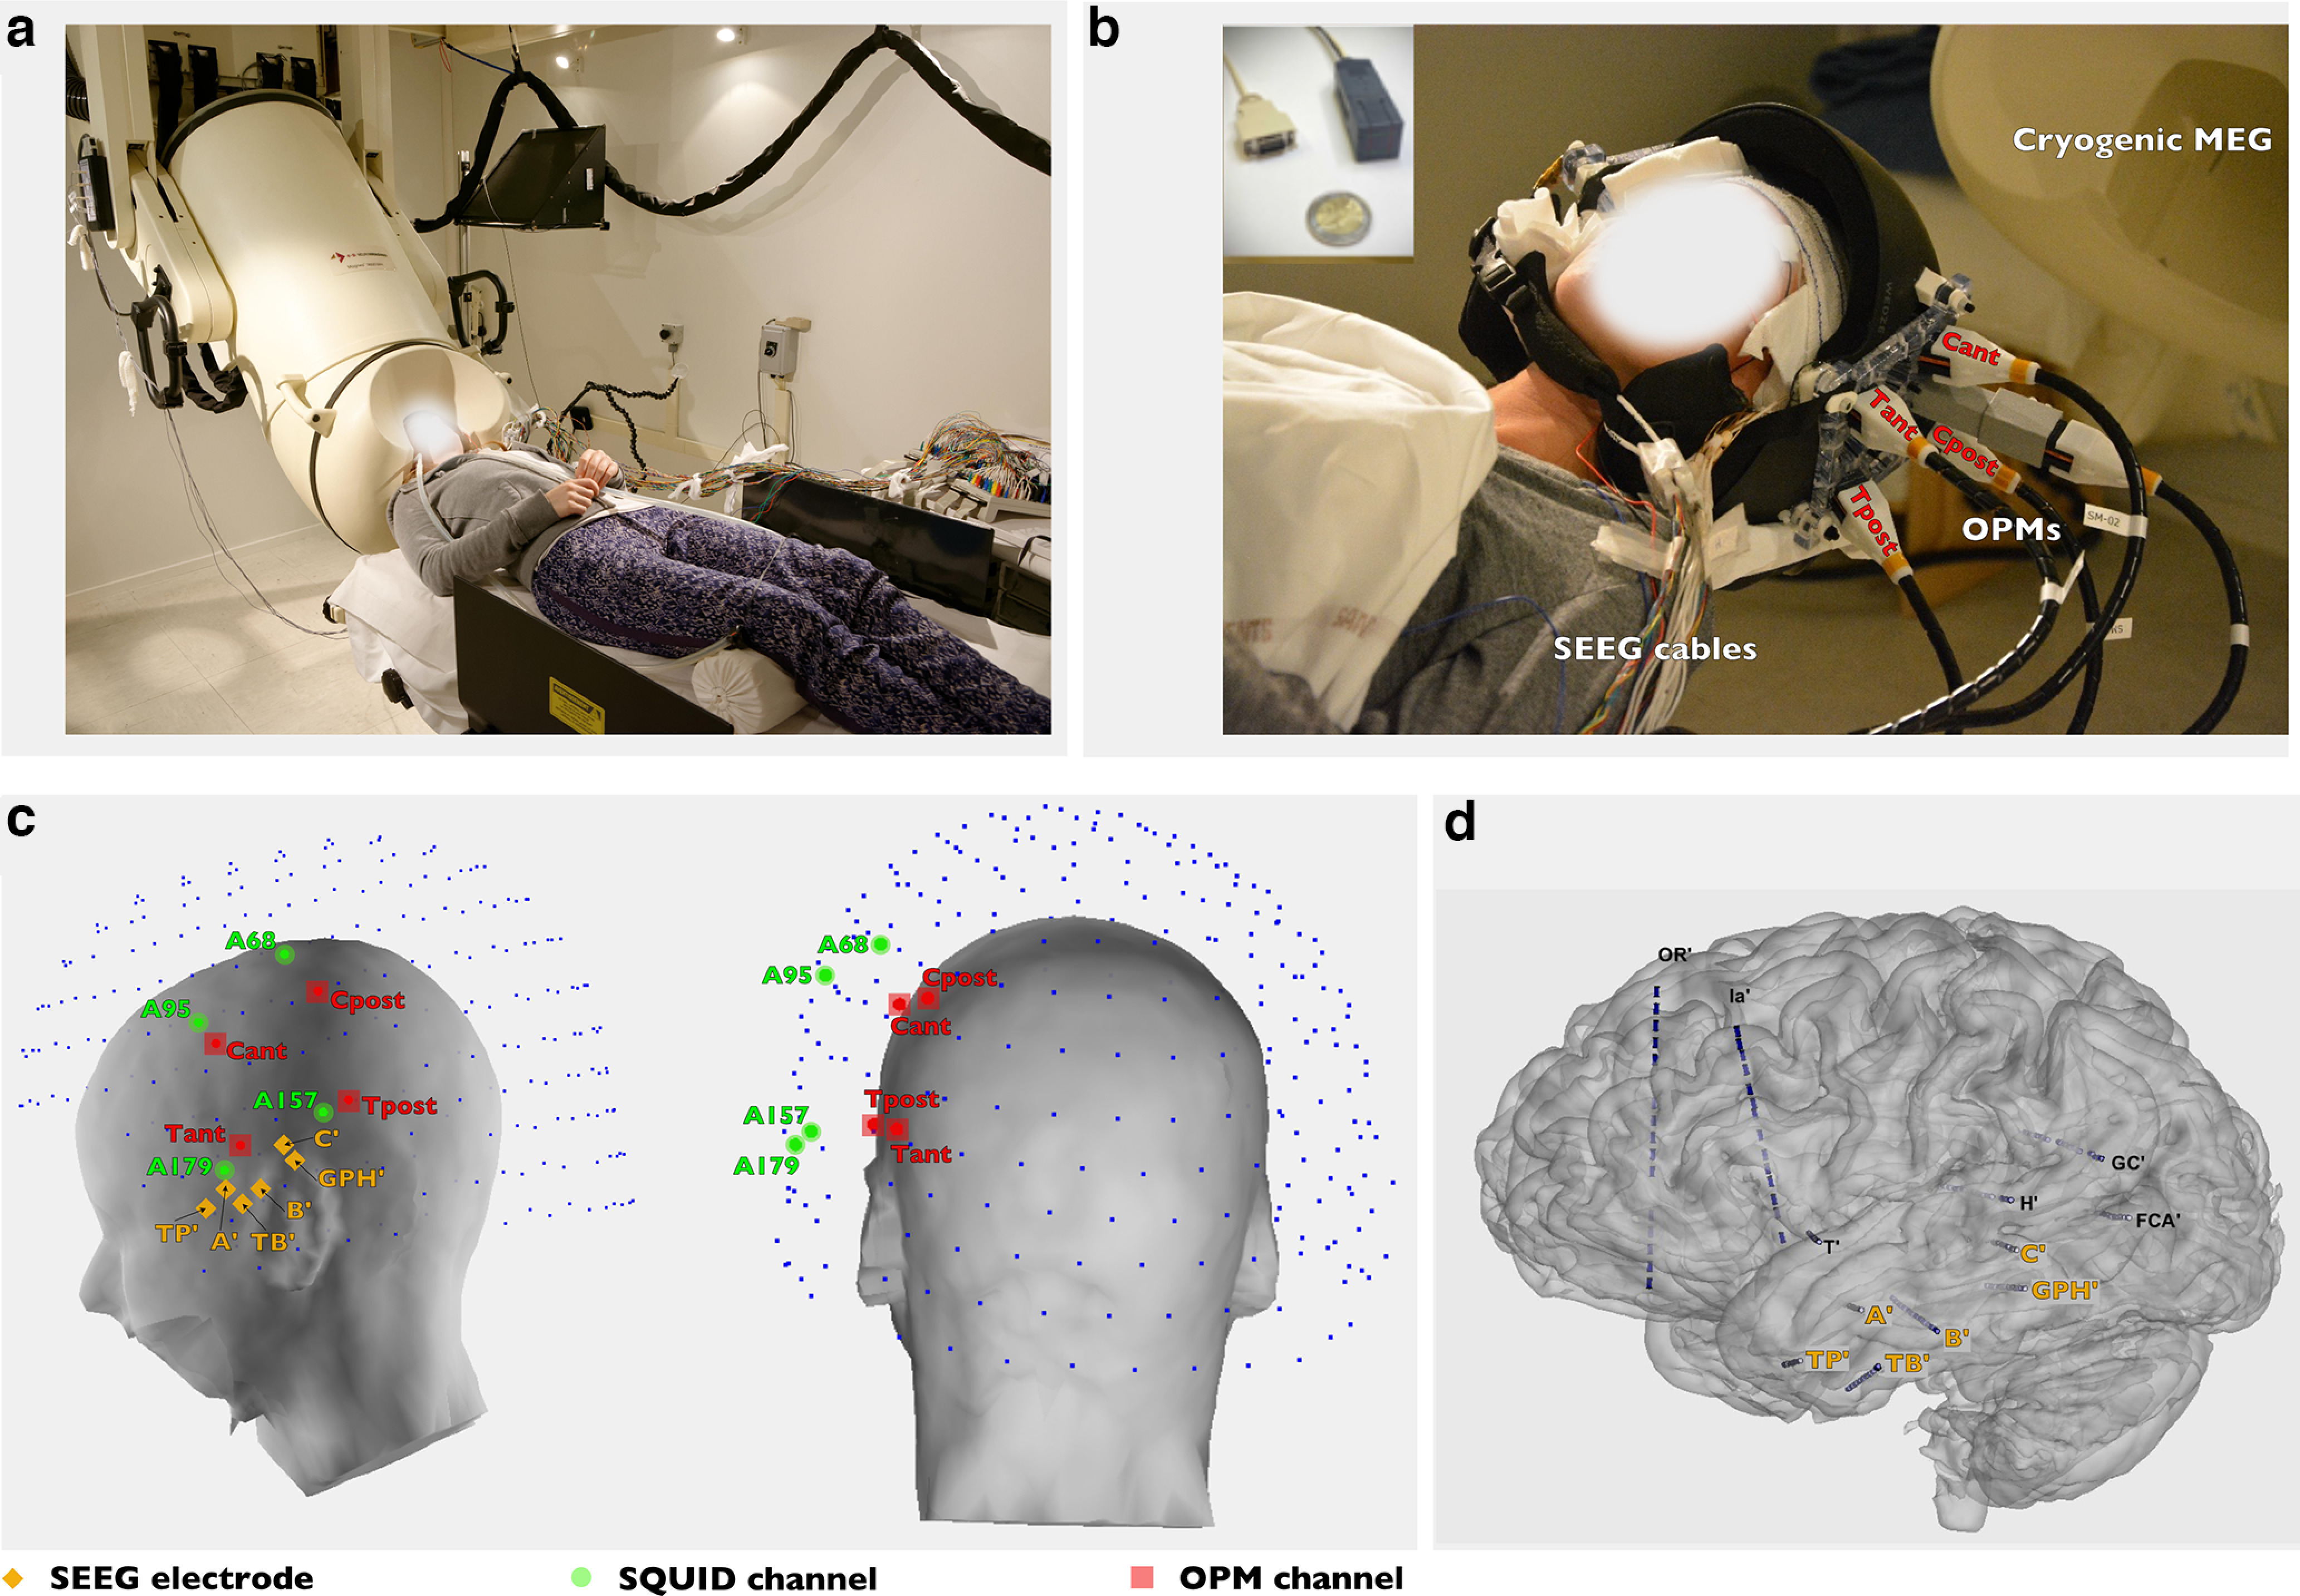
SQUID-MEG, ^4^He-OPM-MEG, and SEEG recording setup. *a*, Simultaneous SQUID-MEG/SEEG. The classic cryogenic MEG system, measuring 120 × 100 cm and weighing ∼300 kg with 265 SQUID sensors in a fixed array requiring subject immobility during data acquisition. *b*, Simultaneous ^4^He-OPM-MEG/SEEG: recording configuration composed of four sensors integrated into a wearable helmet placed on the scalp and in contact with the bandage covering the SEEG electrode inserts (the cables and connectors outside the helmet are visible). Insert, A photo of the ^4^He-OPM sensor. *c*, Three-dimensional reconstruction of the patient's head from MRI, with ^4^He-OPM (red, named Cant, Cpost, Tant, Tpost), SQUID sensors (blue), and SEEG electrodes entry points (orange). The four SQUID sensors (named A95, A68, A179 and A157) closest to the OPMs are in green. Note the distance between the SQUIDs and the scalp (at least 3 cm), whereas the ^4^He-OPMs are in contact with it. *d*, SEEG implantation consists of 14 intracerebral electrodes, 2 in the right hemisphere (not shown) and 12 in the left hemisphere exploring the whole left temporal structures. A’, Amygdala; TB’, rhinal cortex; C’, posterior hippocampus; GPH’, parahippocampal gyrus; T’, anterior insula/lateral T1; H’, thalamus/Heschl gyrus gyrus; Ia’, anterior insula/F2; FCA’, lingual gyrus; GC’, posterior cingulate/T1; OR’, orbitofrontal cortex/middle frontal sulcus.
